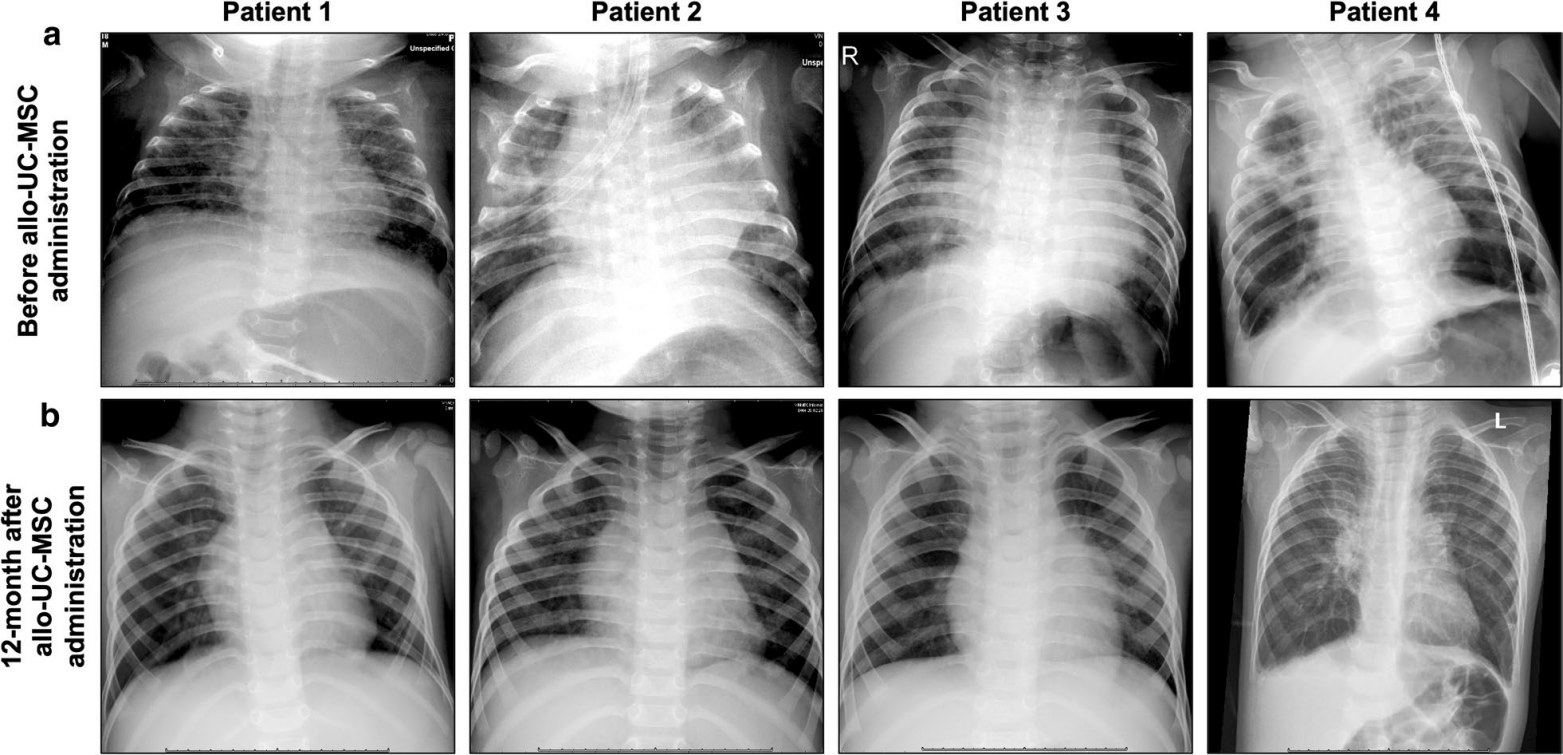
Chest radiographs of the four patients enrolled in the study showing the changes in cystic fibrosis before (a) and 12 months after administration (b). The results indicate the progressive recovery of the lungs, with more air entering both lungs and a reduction in fibrosis after allo-UC-MSC administration
